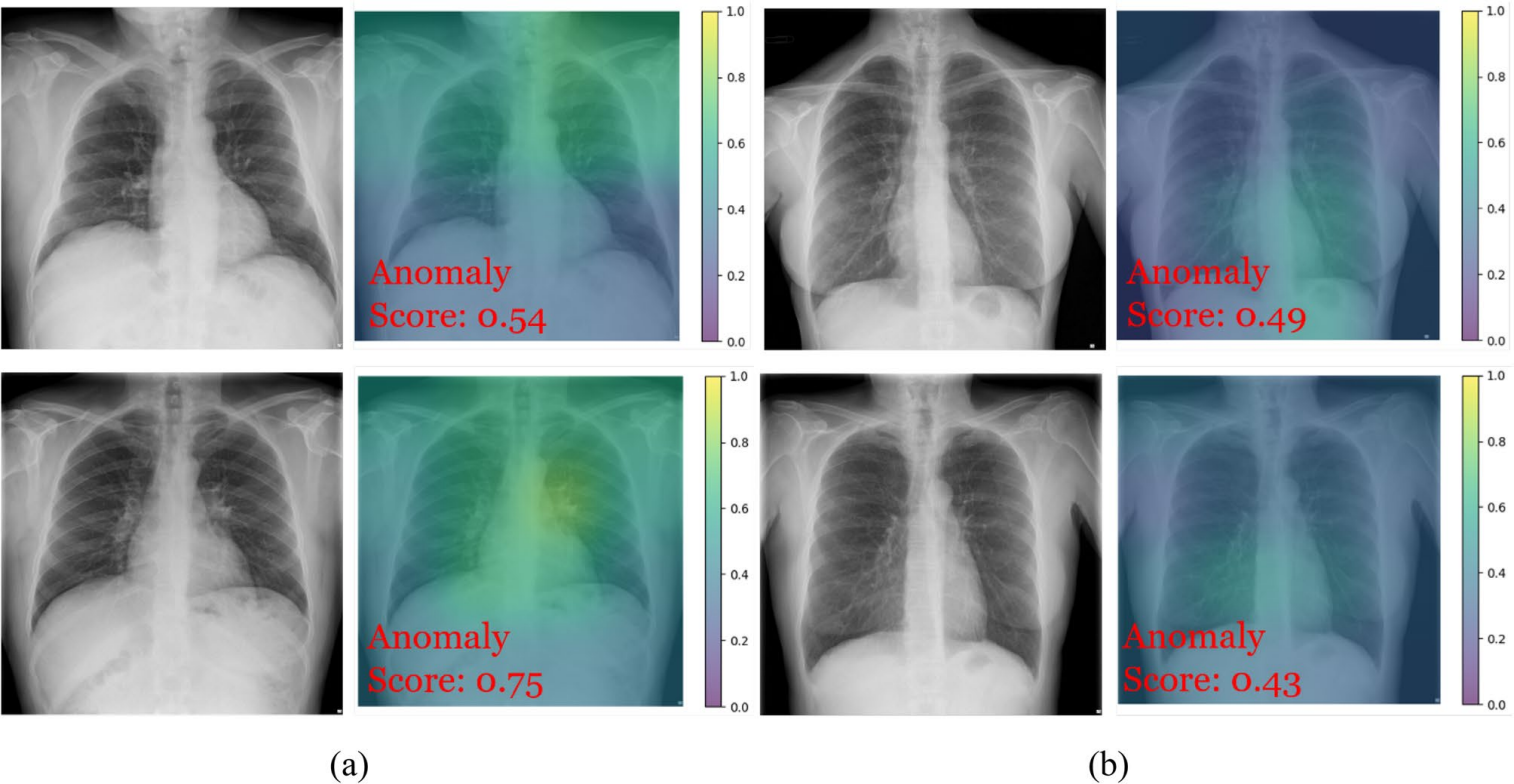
Radiographs of selected misclassified examples—the anomaly scores were generated by the proposed method that showed the best performance in the experiments: (a) Truth label: normal, predicted label: abnormal; (b) truth label: abnormal, predicted label: normal.
